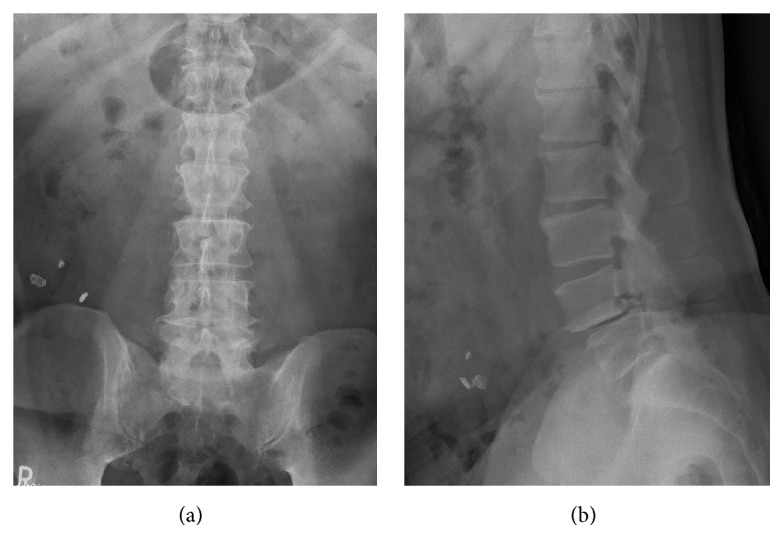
Preoperative radiography of the lumbar spine. Anteroposterior (AP) view (a); lateral view (b). Ossification of the yellow ligament (OYL) is seen at L3/4 and anterior spondylolisthesis of L4 vertebra, with concomitant ossification of the posterior longitudinal ligament.
